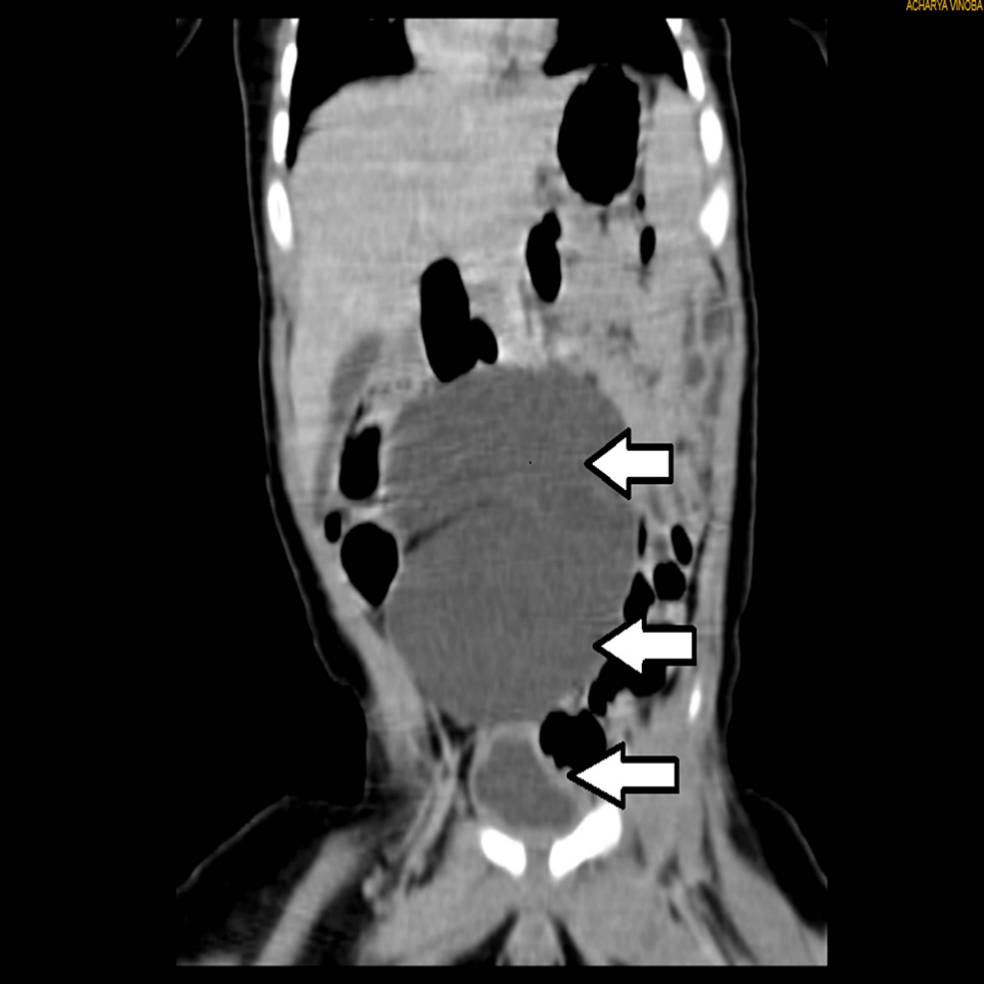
CT urography coronal cut. From top to bottom, the topmost and the middle arrows show a massively dilated renal pelvis with the ureter hardly visualized. The arrow over the bottom shows the bladder in close communication with the renal pelvis.
